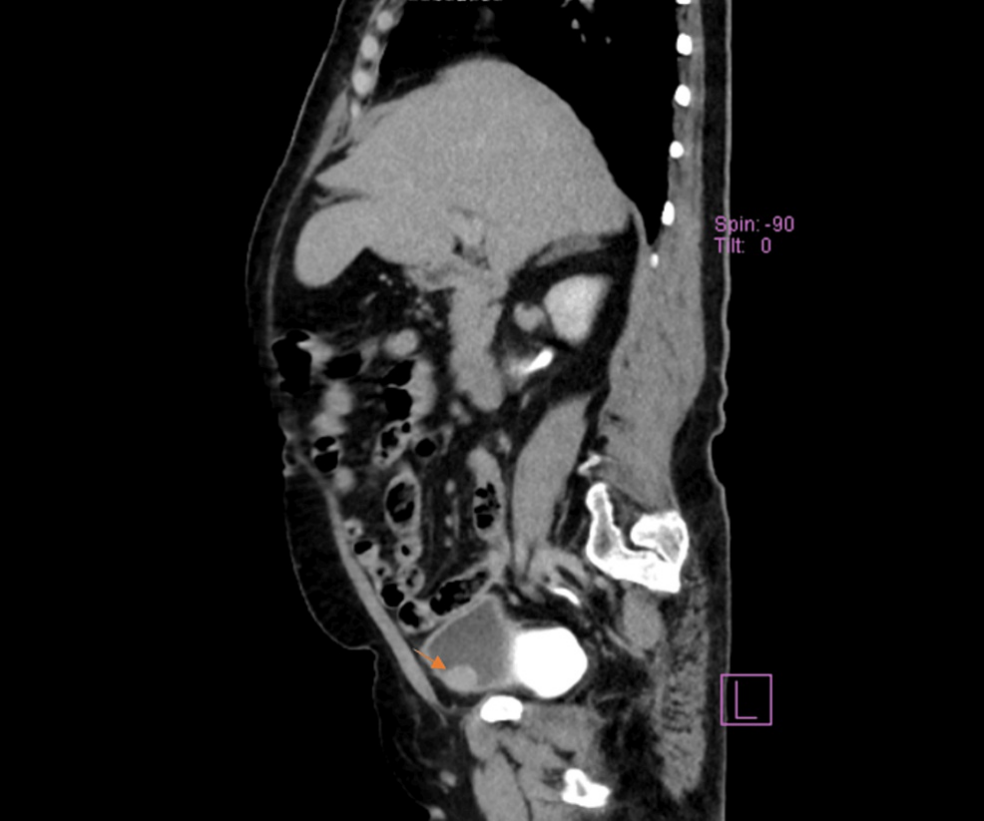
CT abdomen and pelvis without contrast (lateral view) It shows there is a urinary bladder soft tissue lesion seen in the right anterior wall measuring 2 x 1.6 cm in the background of the focal dome wall thickening (arrow). The kidneys are of normal size and show no stones, hydronephrosis, or gross lesions. There is a prominent left inguinal lymph node.
